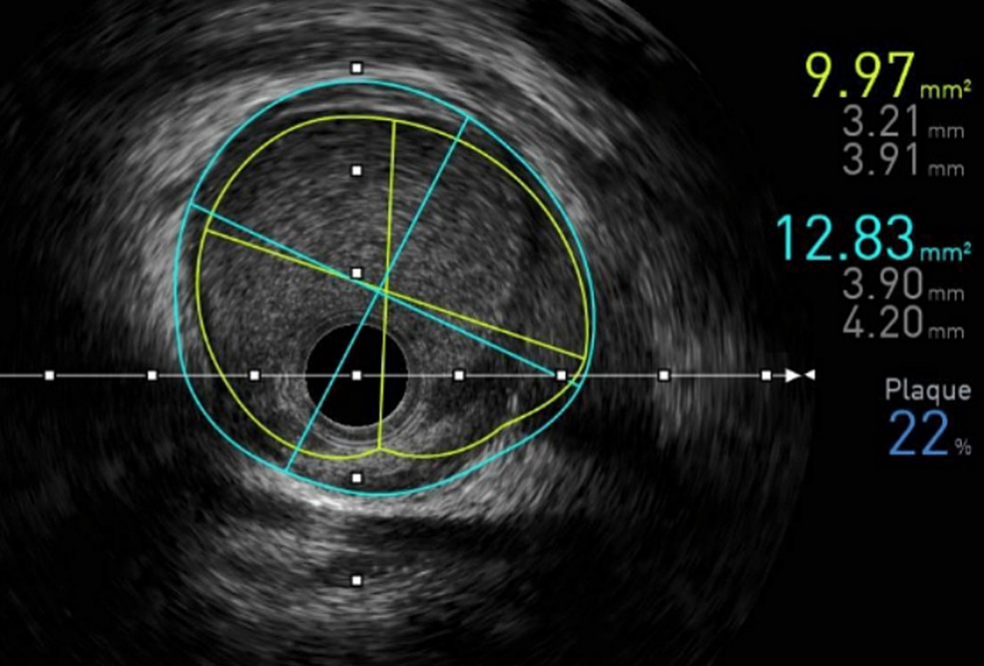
IVUS of the mid-LAD confirming a type I SCAD with a luminal area of 10 mm2. IVUS: intravenous ultrasound, LAD: left anterior descending artery, SCAD: spontaneous coronary artery dissection
